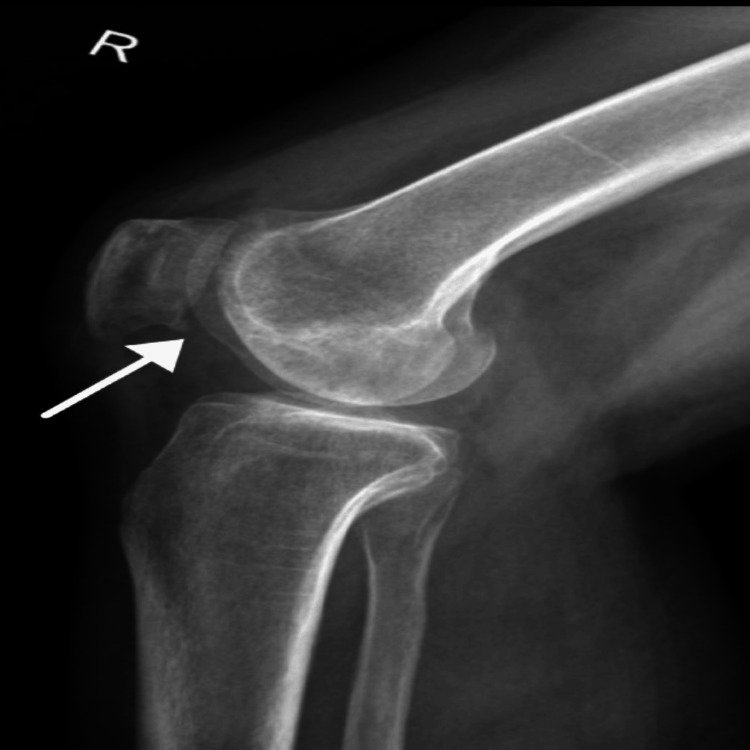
Post-Operative X-ray Findings Lateral view of the left knee after surgical repair The arrows indicate the graft site on the medial femoral condyle.
